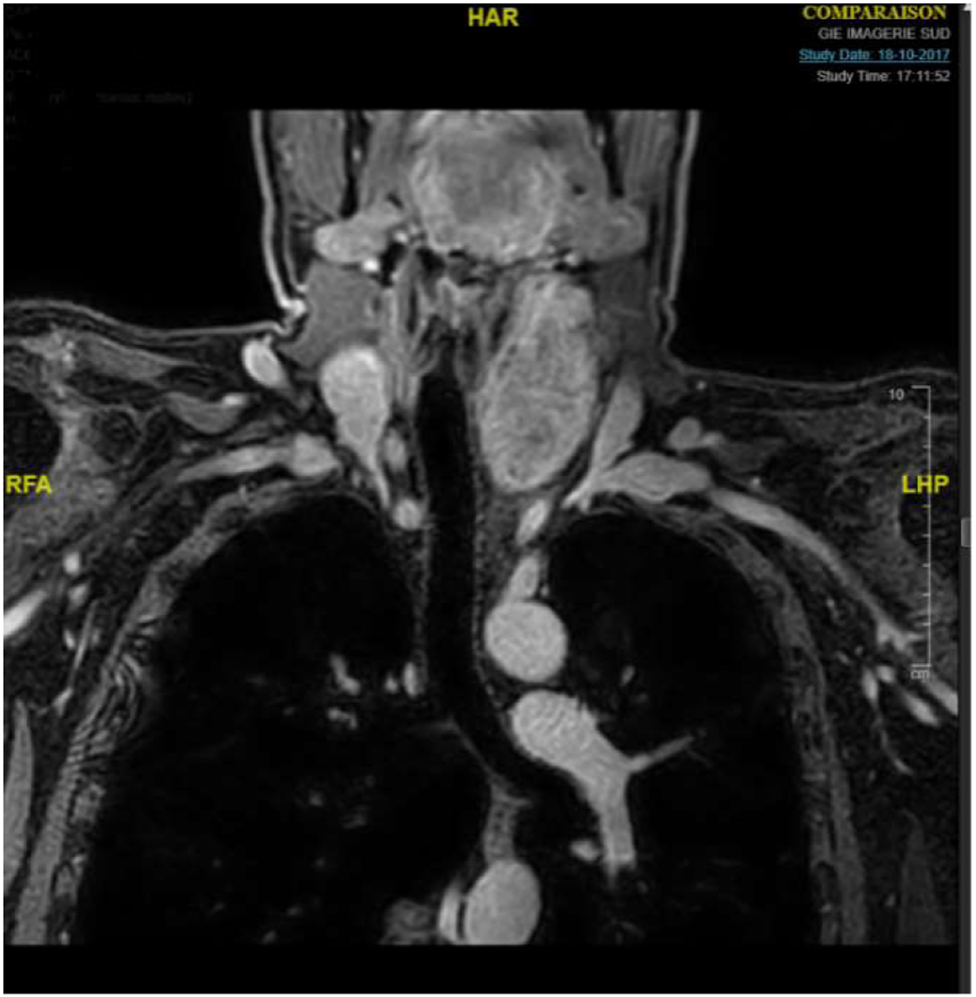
MRI of the left cervical lesion (axial view).
